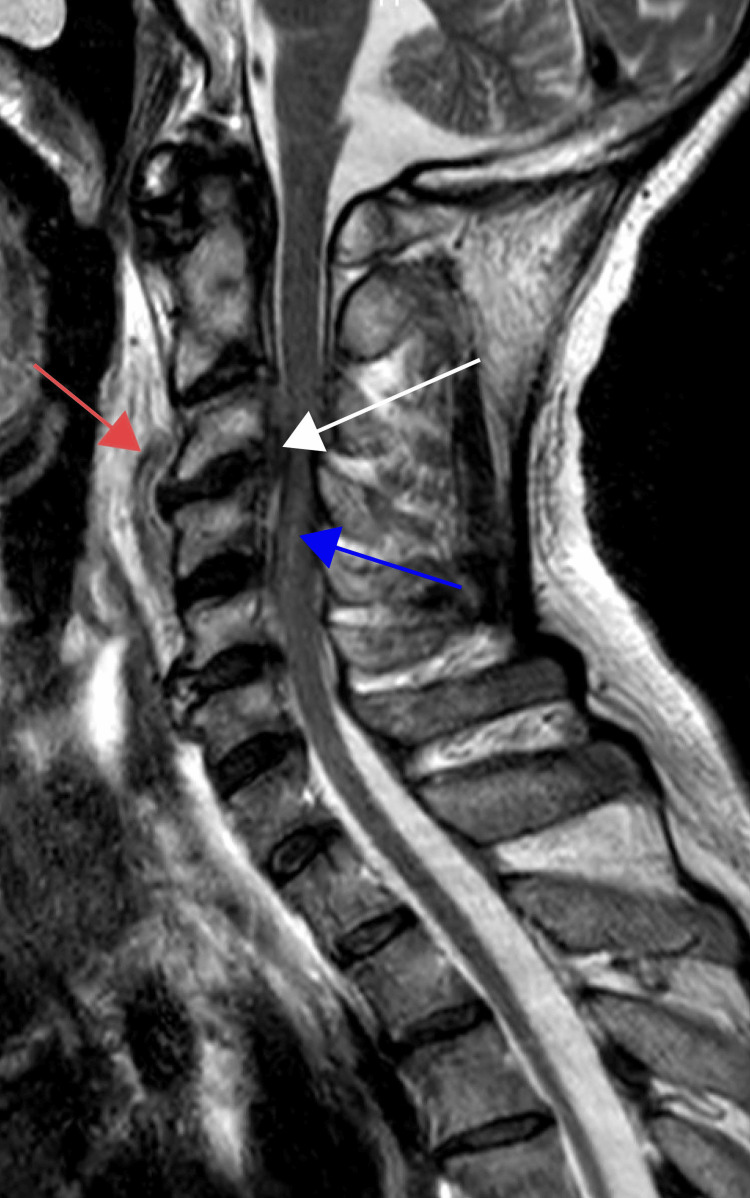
Midsagittal T2-weighted MRI of the cervical spine The image demonstrates extensive C3-C4 spondylodiscitis with significant inflammatory changes. A large prevertebral abscess (red arrow) is visible, extending from C2 to C6 and causing anterior displacement of the retropharyngeal soft tissues. At the C3-C4 level, a critical anterior spinal epidural abscess (white arrow) is seen, leading to severe spinal cord compression with a residual spinal canal diameter of only 5 mm. A diffuse intramedullary T2 hypersignal is present from C3 to C6 (blue arrow), consistent with acute compressive myelopathy.
